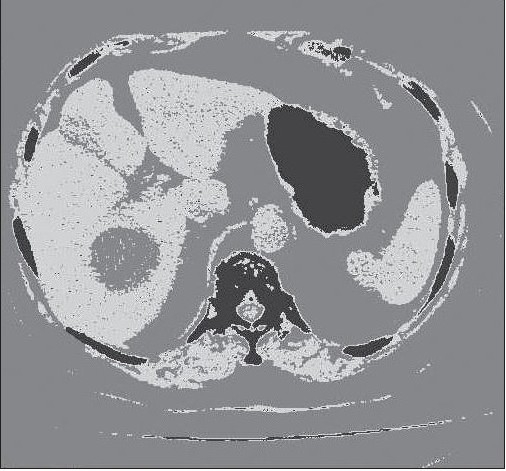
Segmentation of Abdomen (CT image using threshold technique)
